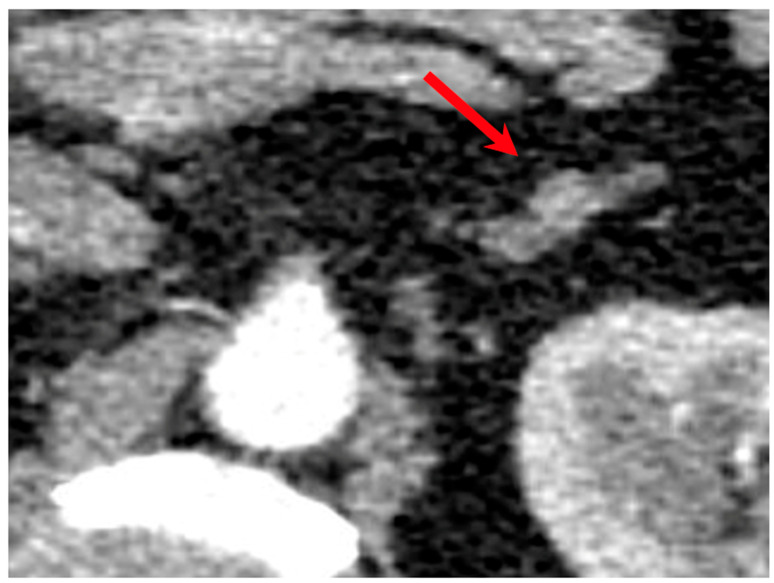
CT scan of an 8 mm left adrenal adenoma. Red arrow indicates the adenoma.
